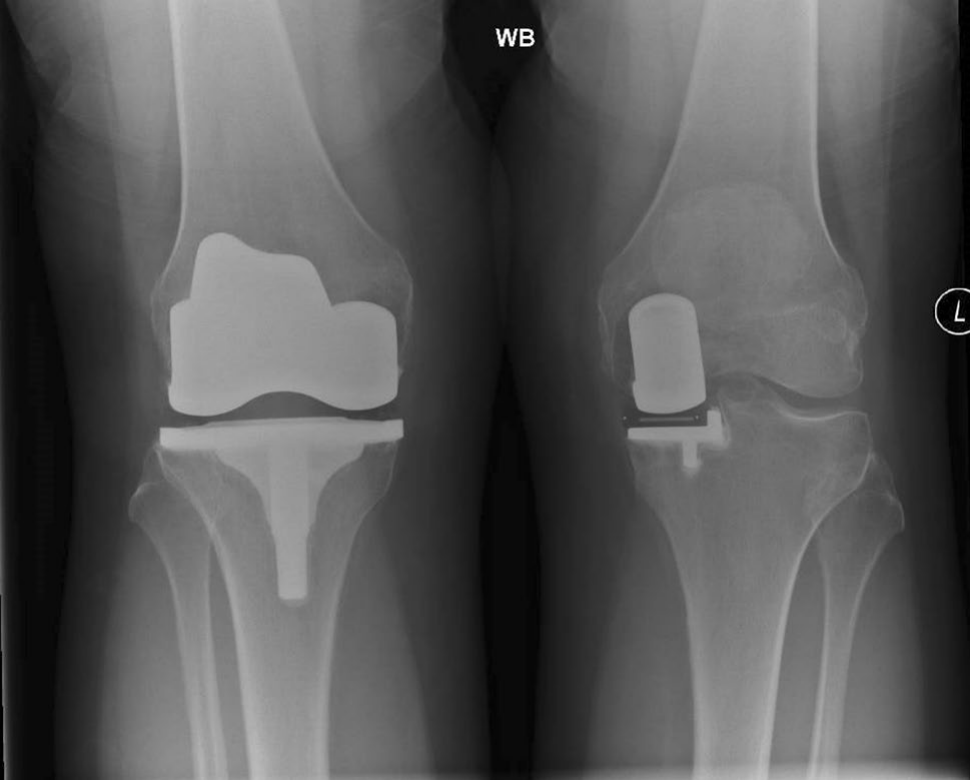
The image shows a postoperative weight bearing plain radiograph after differing knee arthroplasty
